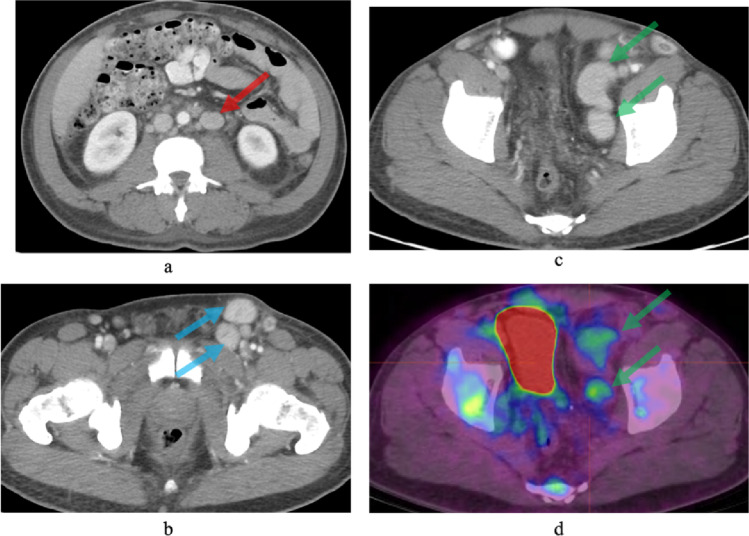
Castleman disease. Axial contrast-enhanced CT images through the retroperitoneum (a) and pelvis (b) show avidly enhancing lymphadenopathy in the left paraaortic (red arrow), left inguinal (blue arrow) and left external iliac (green arrow) chains. The left pelvic lymphadenopathy (green arrow) was FDG-avid on a subsequent PET scan (d). The patient had biopsy-proven Castleman’s disease
